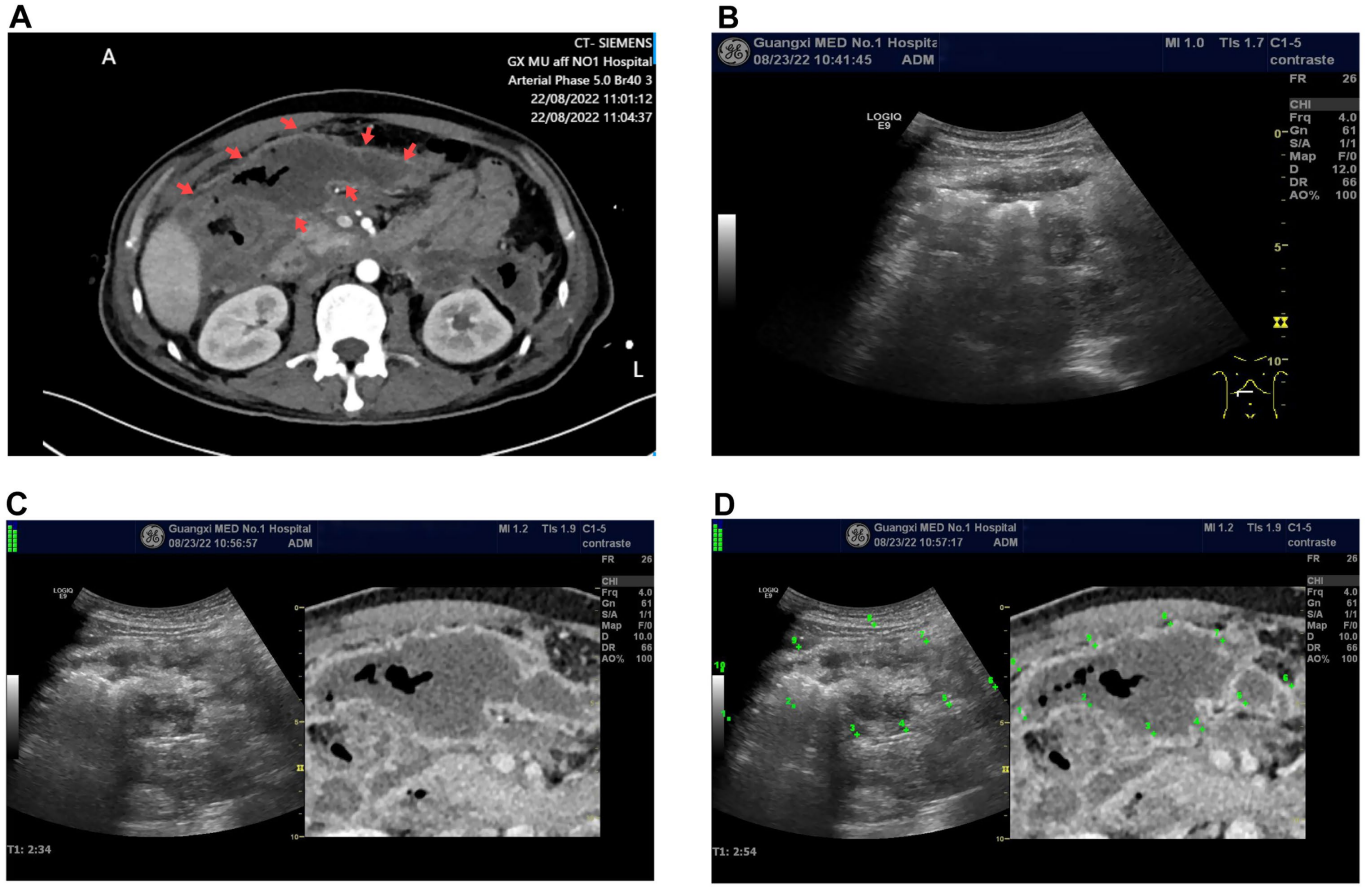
(A) Enhanced CT image of a peripancreatic abscess, where the target lesion appears as a low-density area (indicated by the arrow). It shows no enhancement on the enhanced CT, with gas density shadows inside. (B). The grayscale ultrasound image at the corresponding location of the peripancreatic abscess indicated in the enhanced CT fails to distinguish the lesion. (C) The ultrasound-CT fusion imaging virtual navigation shows the peripancreatic abscess (unmarked), with the grayscale ultrasound image on the left and the enhanced CT image on the right. (D). Point-to-point marking of the peripancreatic abscess as shown in (C), using the enhanced CT delineation of the lesion as a reference, allows the corresponding lesion location to be observed on the ultrasound image.
